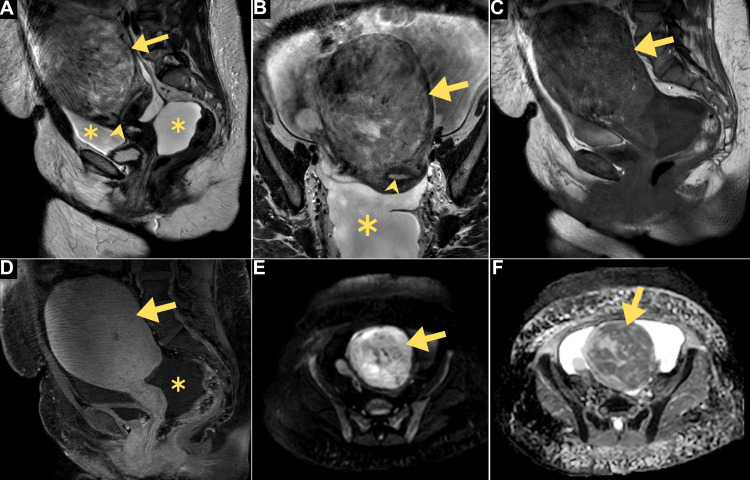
Multiplanar pelvic MR images. (A) Sagittal and (B) coronal T2-weighted images depicting a ventral uterine mass (arrows) expanding the anteverted uterus, compressing the endometrium (arrowhead), and demonstrating predominant tumoral hyperintensity. (C) Sagittal T1-weighted images precontrasted and (D) postcontrasted reveal hypointense signals and homogeneous hyperenhancement. (E) Axial diffusion-weighted imaging showing a hyperintense mass that appeared hypointense on the apparent diffusion coefficient map (F), indicating restricted diffusion. Notably, there is a conspicuous presence of surrounding ascites (denoted by asterisks). MR: magnetic resonance
